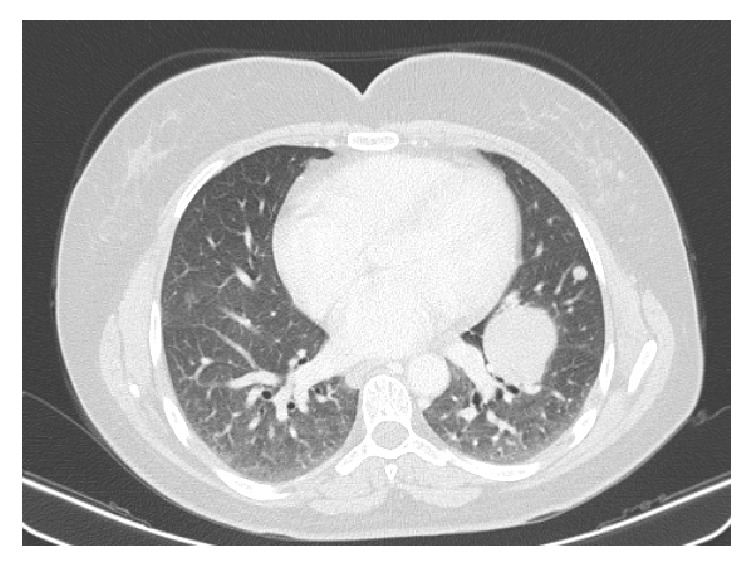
Chest CT, axial image. There is a soft tissue density mass measuring 44 mm and an 8 mm nodule, both in the left lung.
